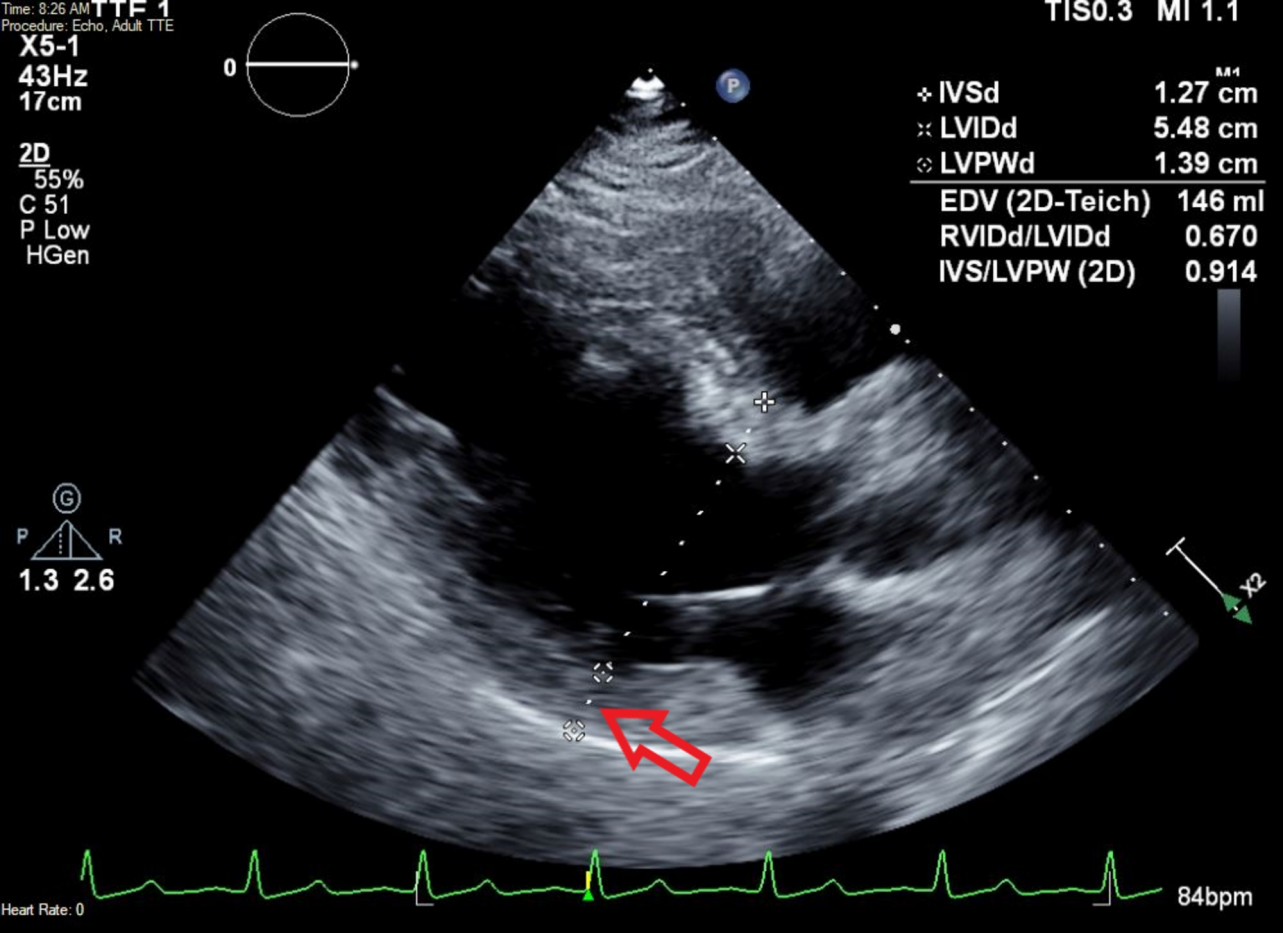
Echocardiogram (parasternal long-axis view) showing thickened end-diastolic left ventricle posterior wall (red arrow).
